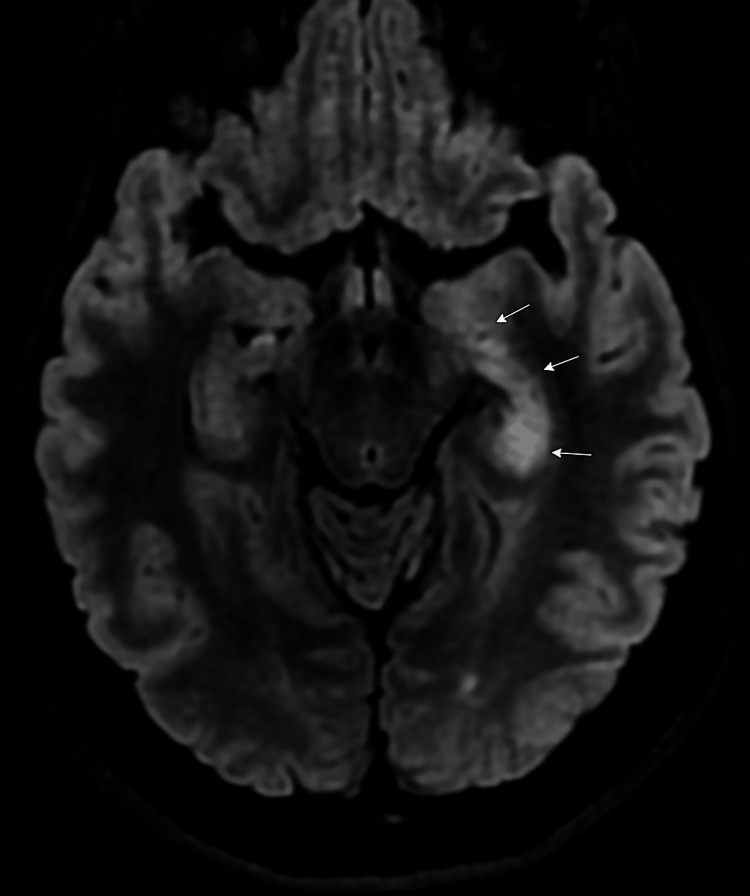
Brain magnetic resonance imaging, axial FLAIR sequences showing increase signal within the left mesiotemporal lobe (arrows), 2018 FLAIR: fluid attenuated inversion recovery
